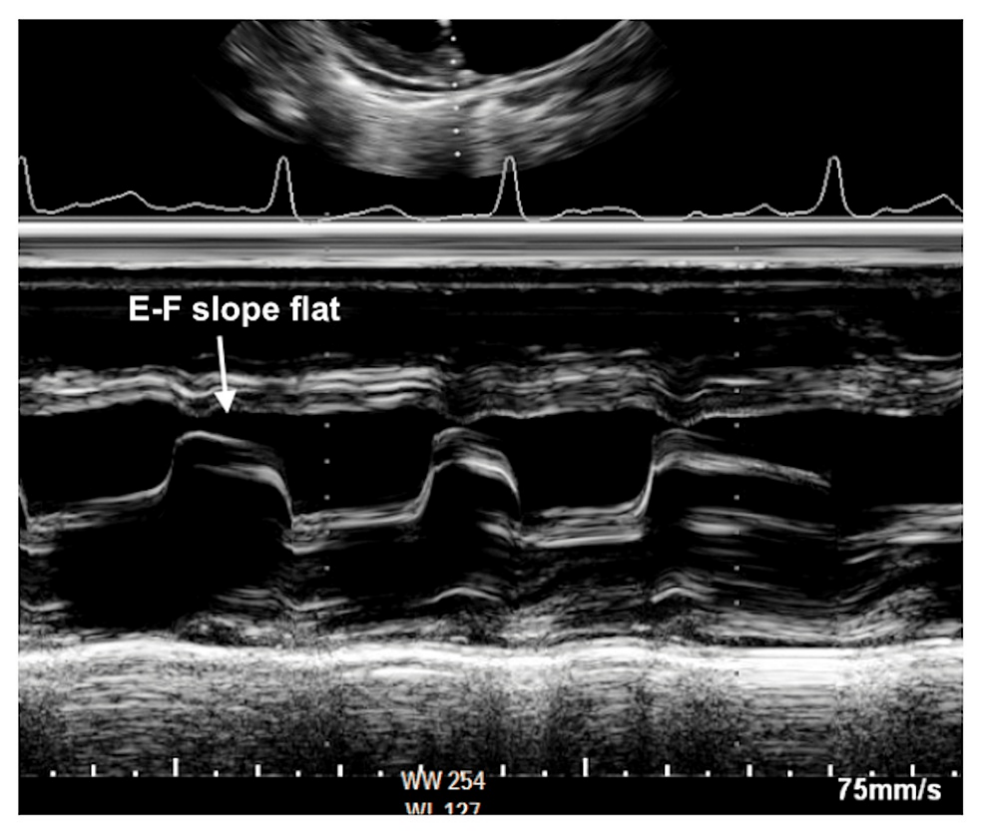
Transthoracic echocardiography M-mode showing E-F slope flattening
